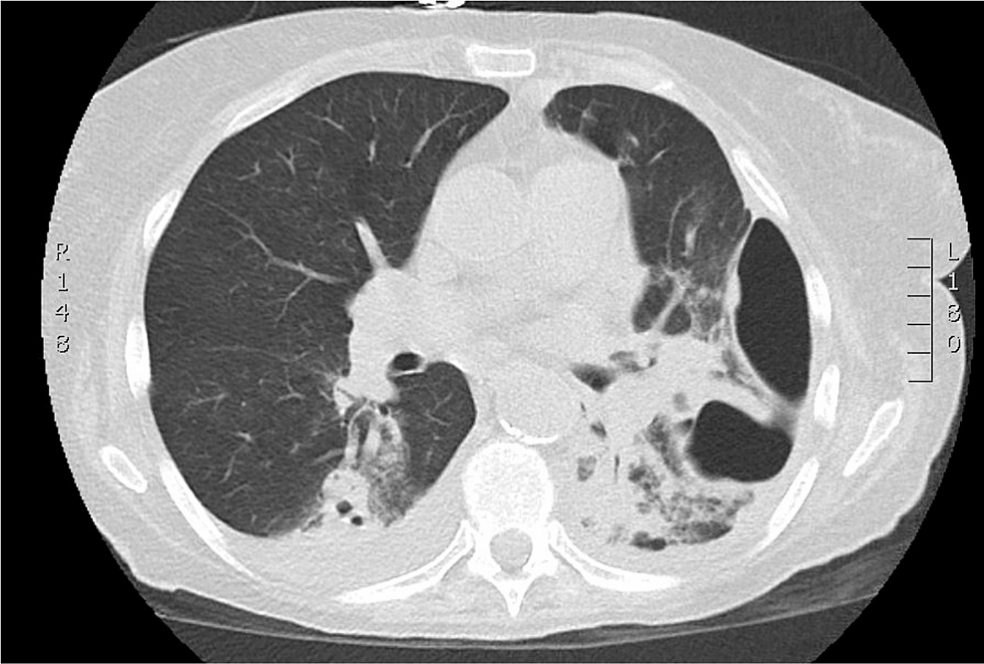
CT chest (axial view) of patient’s necrotizing pneumonia with empyema, complicated by persistent pneumothorax and bronchopulmonary fistula.
